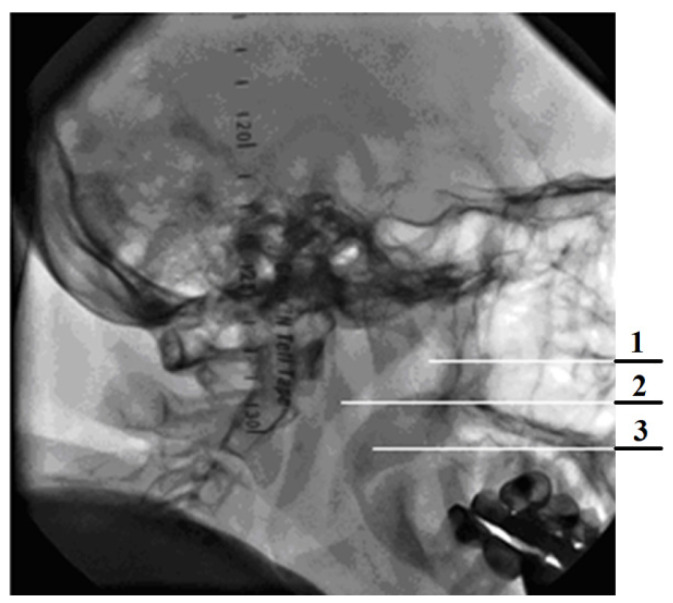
Static image of a frame of high-speed dynamic radiography of the nasopharynx in a sagittal projection (1—airway of the nasopharynx, 2—posterior pharyngeal wall, 3—soft palate).
